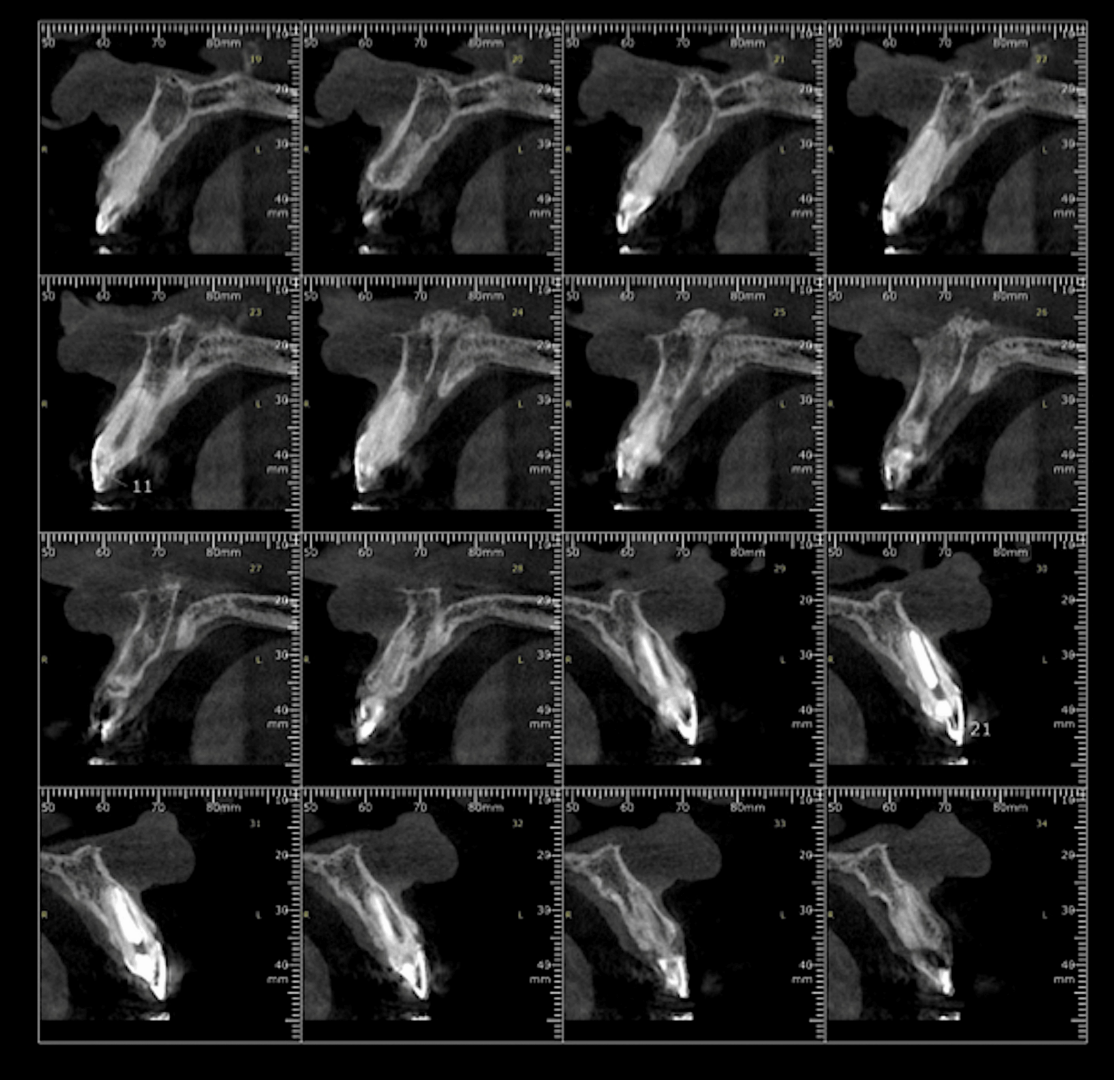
Cone-beam computed tomography analysis of the upper anterior sextant.
